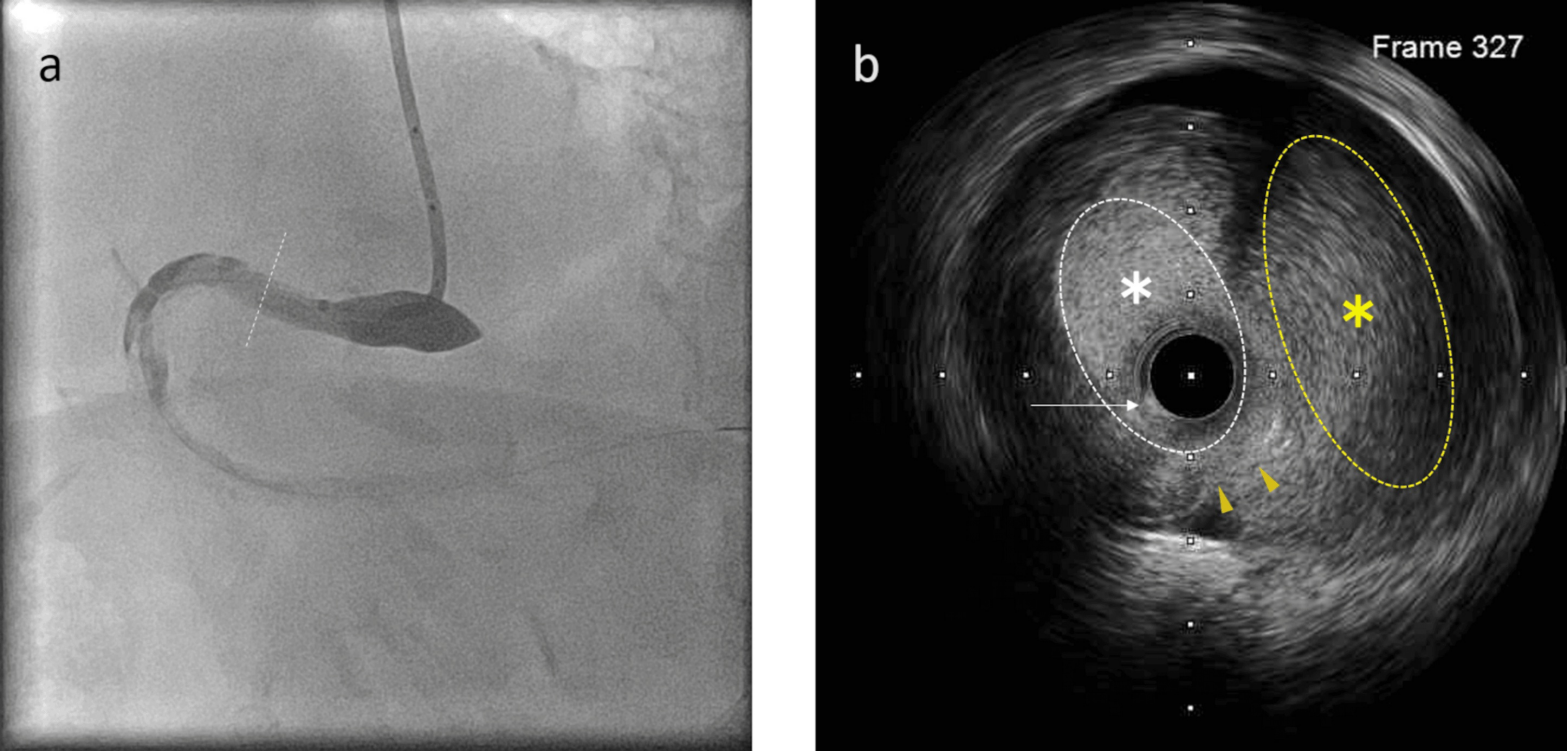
Right coronary angiography and cross-sectional intravascular ultrasound image. a: The white dotted line in the right coronary angiography indicates the location of the corresponding cross-sectional intravascular ultrasound image. b: Cross-sectional intravascular ultrasound image showing the true lumen (white asterisk) and pseudo-lumen (yellow asterisk). The guidewire is located in the true lumen (white arrow). Intimal dissection is also visible (orange arrowheads).
